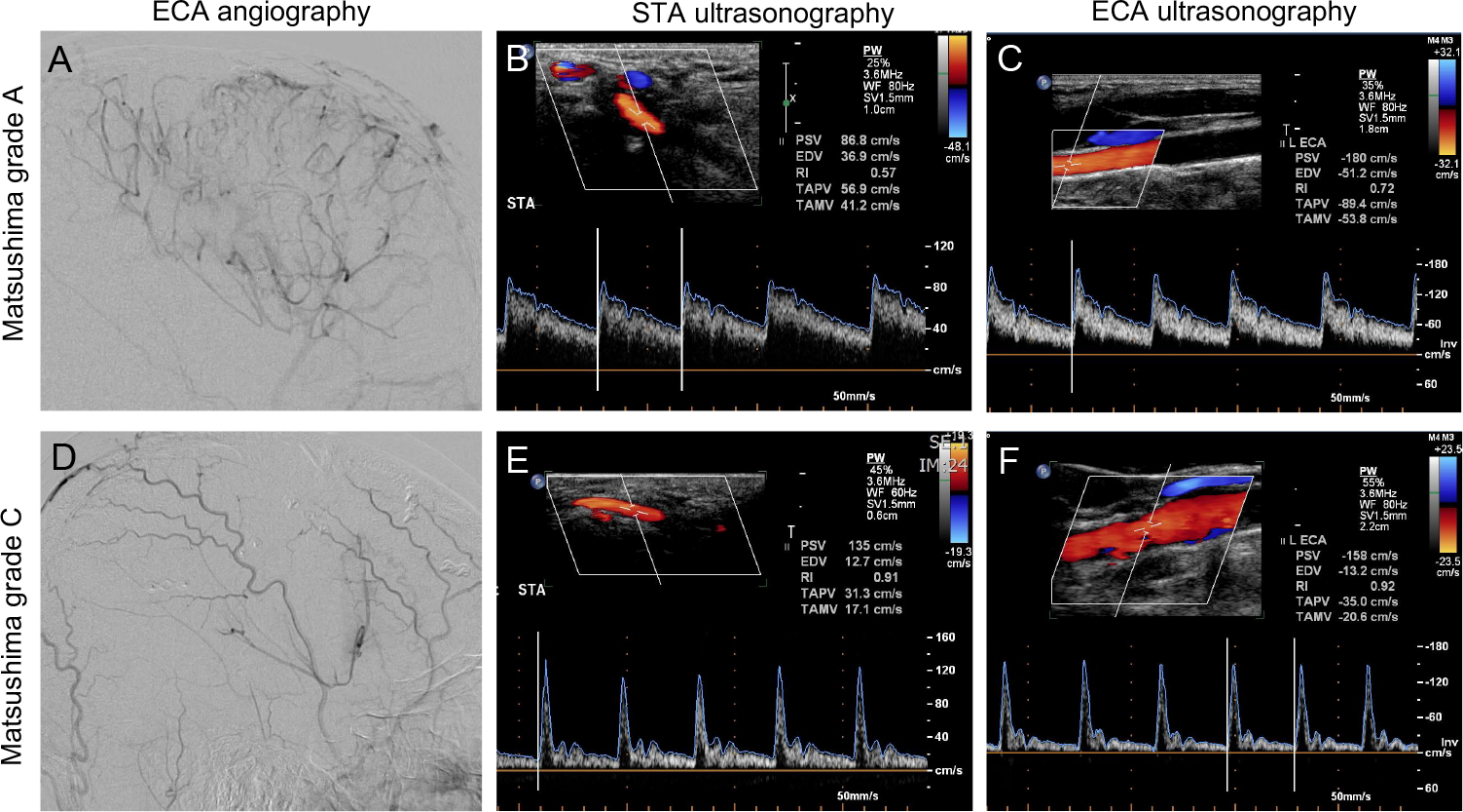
Postoperative angiogram and ultrasonographic flow patterns of ipsilateral STA and ECA in two representative cases. Case 1: Well-developed collateralization on ECA angiogram (Fig 1A) and reduced resistance in the STA (Fig 1B) and ECA (Fig 1C) on ultrasound. Case 2: Poor collateral establishment on ECA angiogram (Fig 1D) and ultrasound showed high resistance pattern in the STA (Fig 1E) and ECA (Fig 1F).
